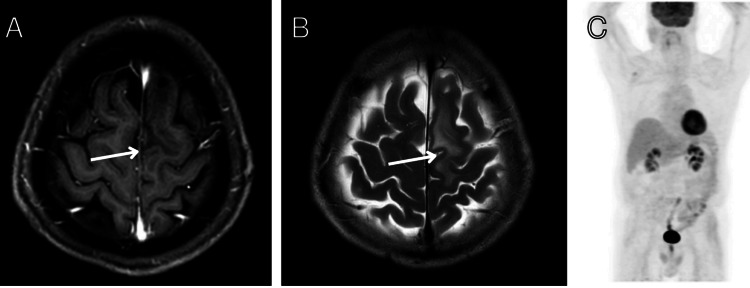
Complete response to combination therapy. (A) Axial T1-weighted contrast-enhanced MRI brain image demonstrating complete response of the dural metastasis four months after initiation of combination osimertinib and alectinib therapy. (B) Axial T2-weighted MRI brain image demonstrating near complete resolution of edema four months after initiation of combination osimertinib and alectinib therapy. (C) Whole body PET-CT demonstrating complete extracranial response four months after initiation of combination osimertinib and alectinib therapy, showing no evidence of metabolically active disease.
